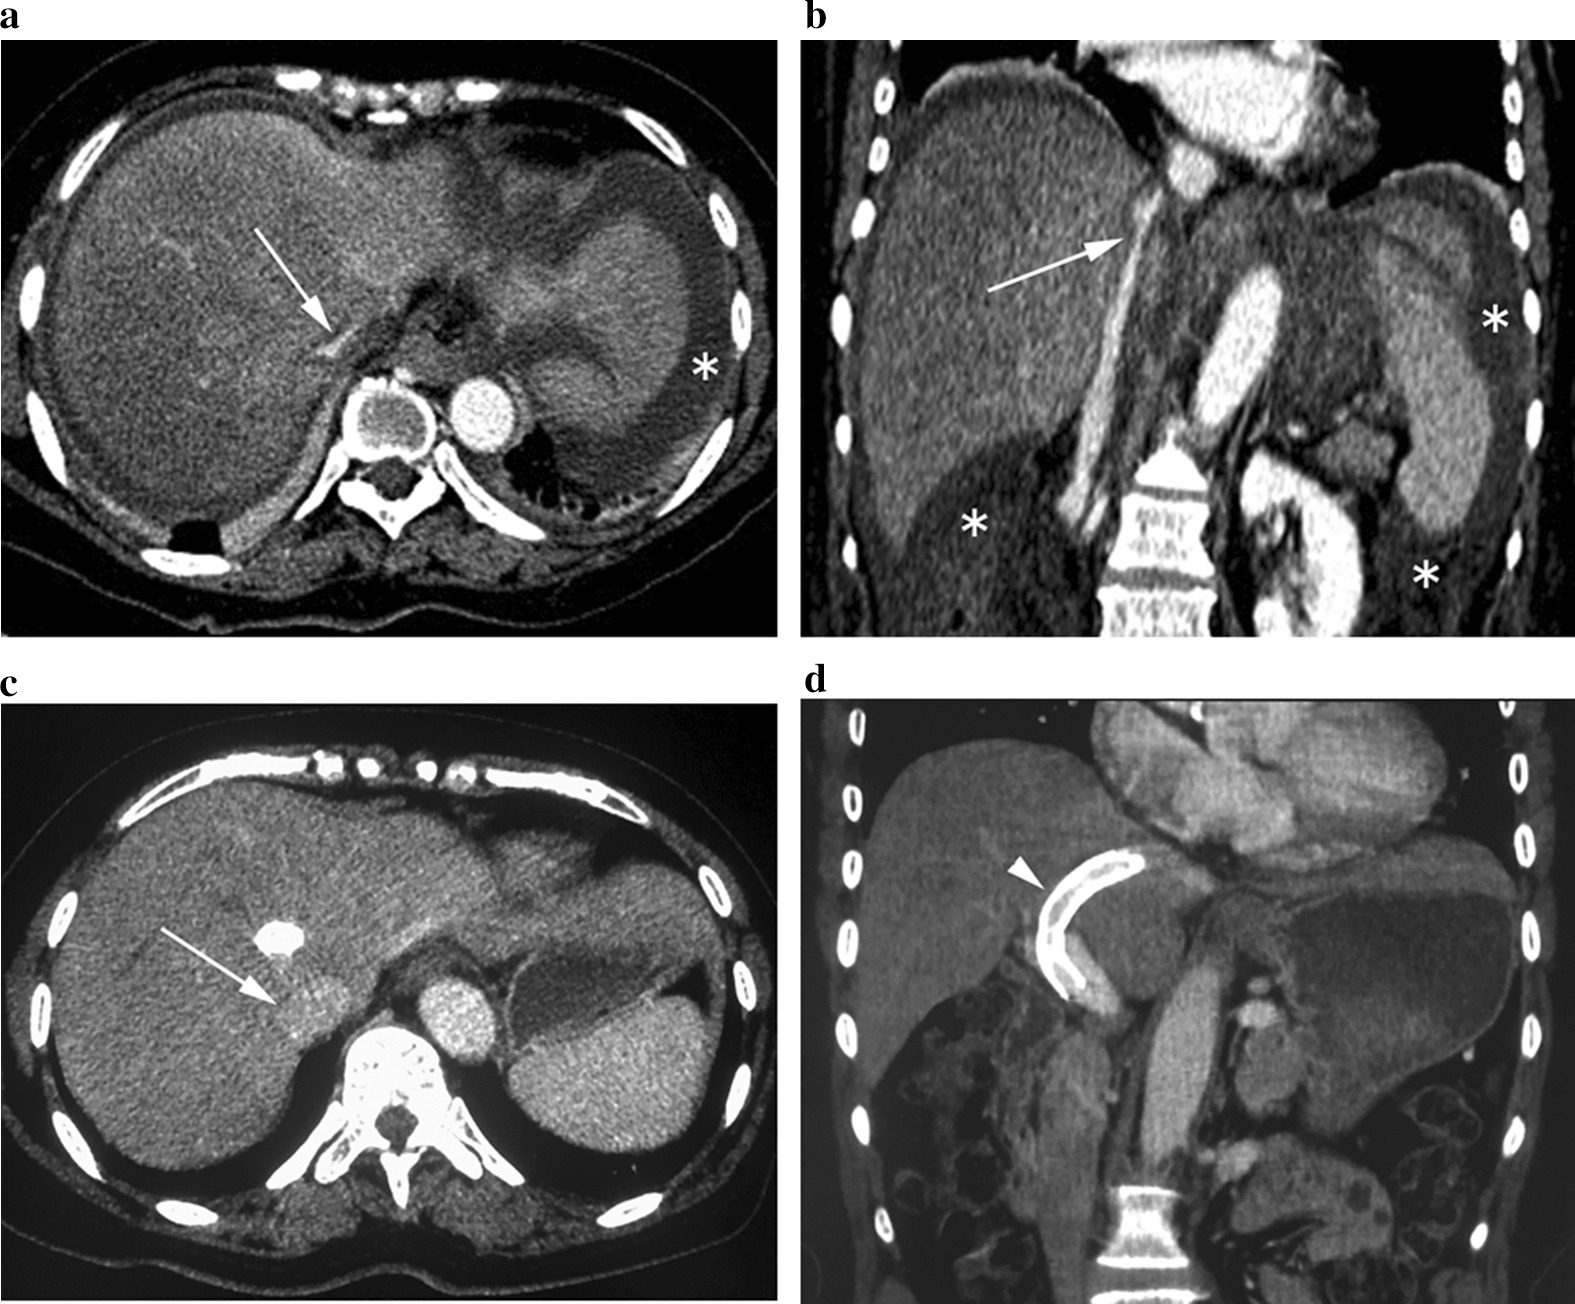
CT findings of GS-related HSOS before and after TIPS. Contrast-enhanced axial (a) and coronal (b) CT images showed patchy heterogeneous enhancement of the liver, with increased size of the liver, decreased size of the hepatic veins and hepatic segment of the inferior vena cava (arrow), and massive ascites (asterisk). Follow-up axial (c) and coronal (d) CT images demonstrated that the liver enhancement returns to homogeneous, with normal size of the liver, hepatic veins and inferior vena cava (arrow), and complete resorption of ascites. Note the patent TIPS stents (arrowhead)
